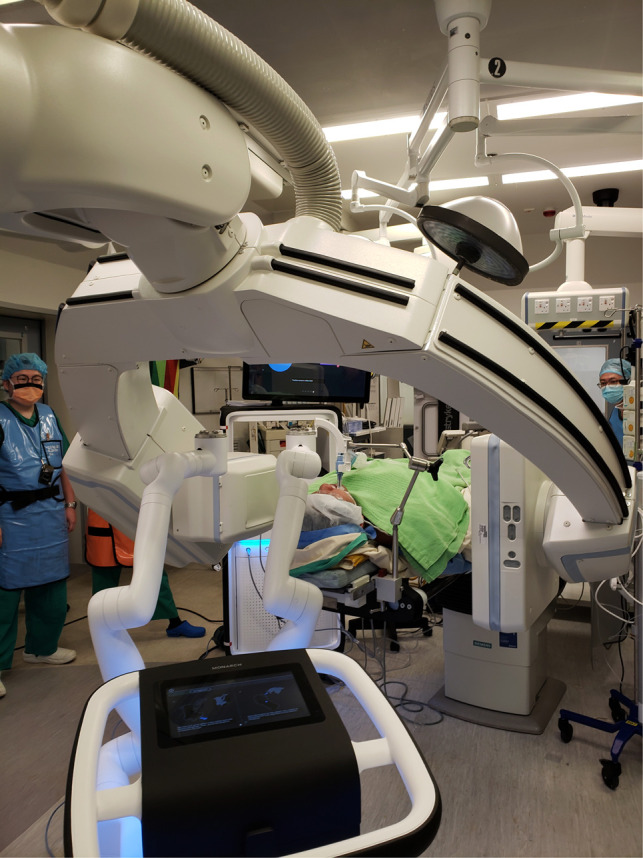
The left robotic arm can be seen in very close proximity to the cone-beam CT (CBCT) C-arm during CBCT spin. The metallic holder of the endotracheal tube is also close to the other side of the CBCT C-arm. Care must be taken to avoid collisions in these two regions.
